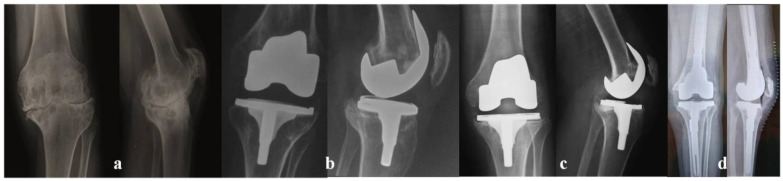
A 34-year-old and heavy worker patient with severe arthropathy of his right knee—Pettersson score 11 (a), undergoing a posterior stabilized TKA. X-rays at 1 year (b) and 13 years (c) after surgery. A symptomatic aseptic loosening was demonstrated, and the patient underwent a revision with an oxidized zirconium femoral component, titanium tibial component, and posterior stabilized high-flexion PE insert with cementless stems (d).
